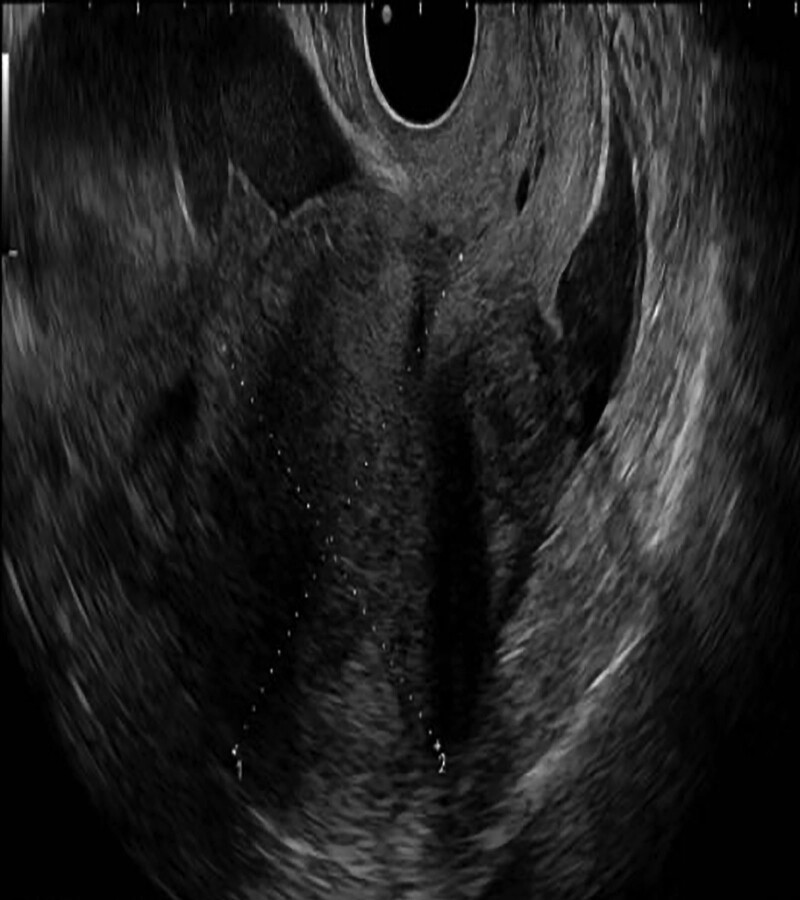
Ultrasound shows a fetal echo above the uterus in the abdominal cavity.
